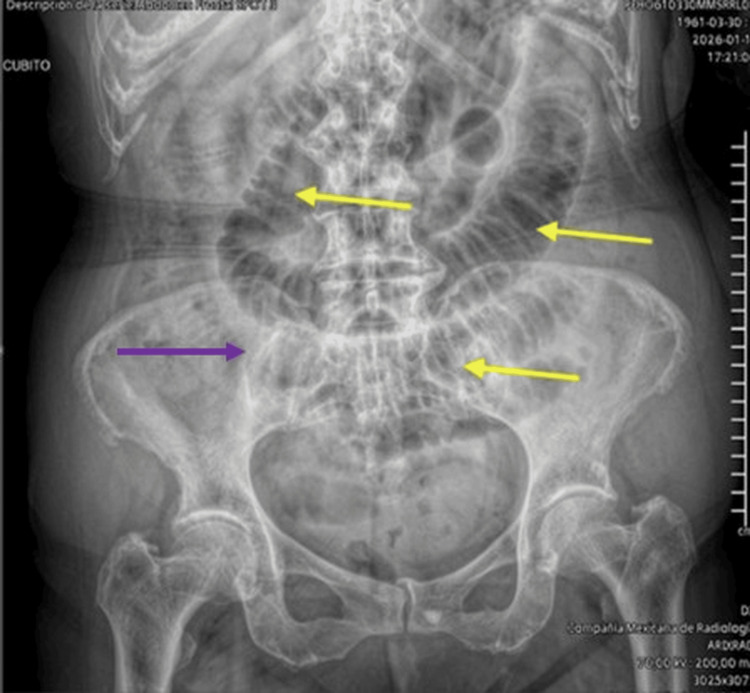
Supine X-ray showing dilated small bowel loops with a “stack of coins” appearance (yellow arrows) and a strangulated bowel loop within a Spigelian hernia (purple arrow), suggestive of mechanical small bowel obstruction.
